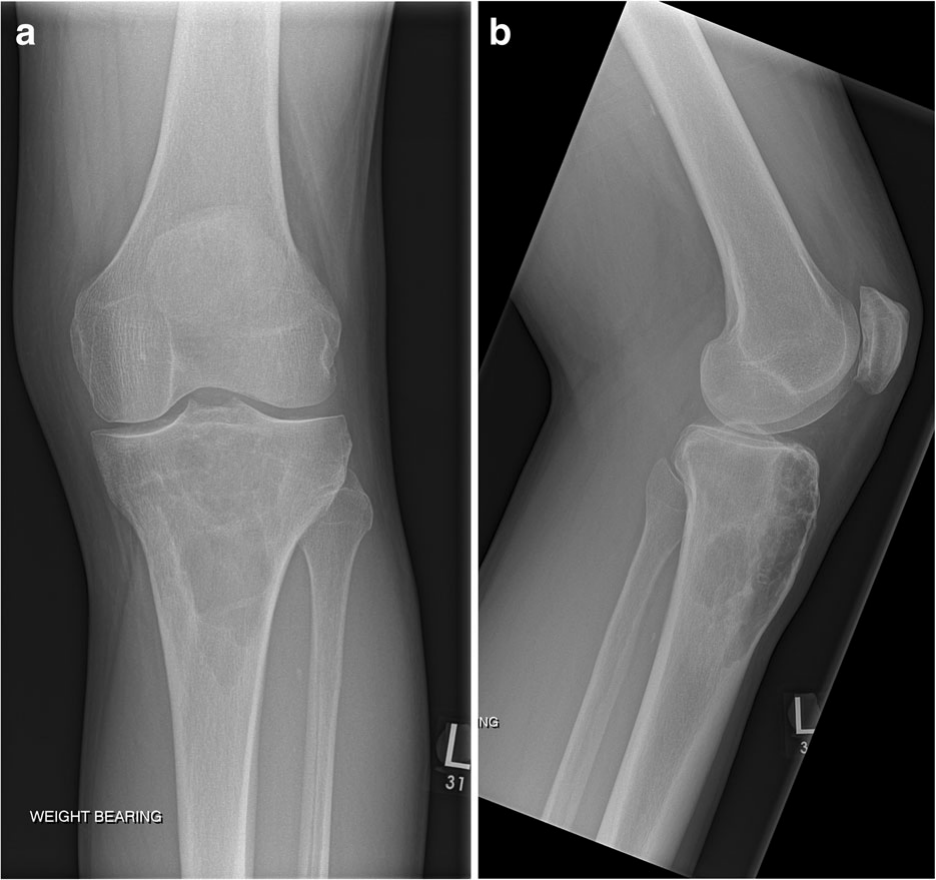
a, b The plain film of his left knee showed a multiloculated lytic lesion in the proximal tibial metaphysis close to the cortical surface
